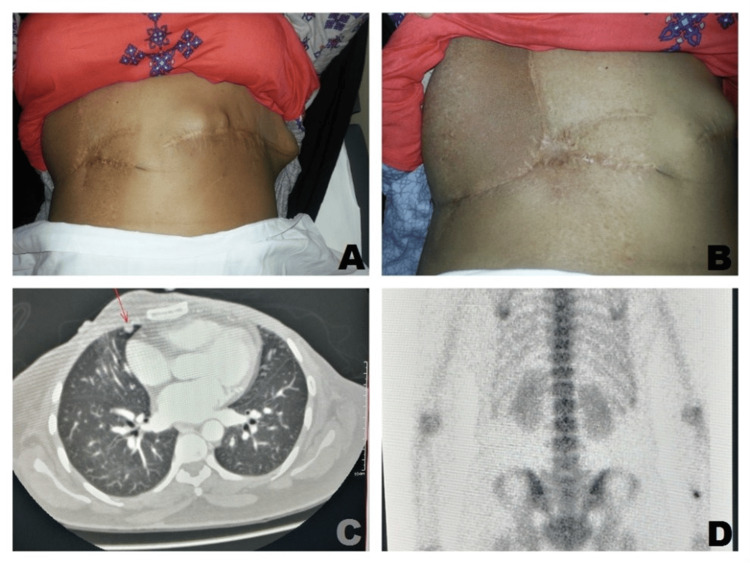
(A) Bilateral mastectomy scars showing new lumps on the left side. (B) Latissimus dorsi flap reconstruction in the right axilla. (C) CT showing metastasis to the lung. (D) Bone scan with rib metastasis
